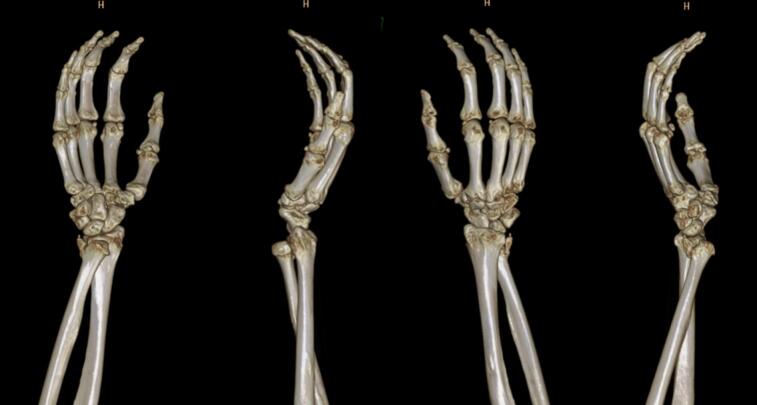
Wrist 3D CT-Scan revealed a volar DRUJ dislocation with an isolated ulnar styloid fracture.
